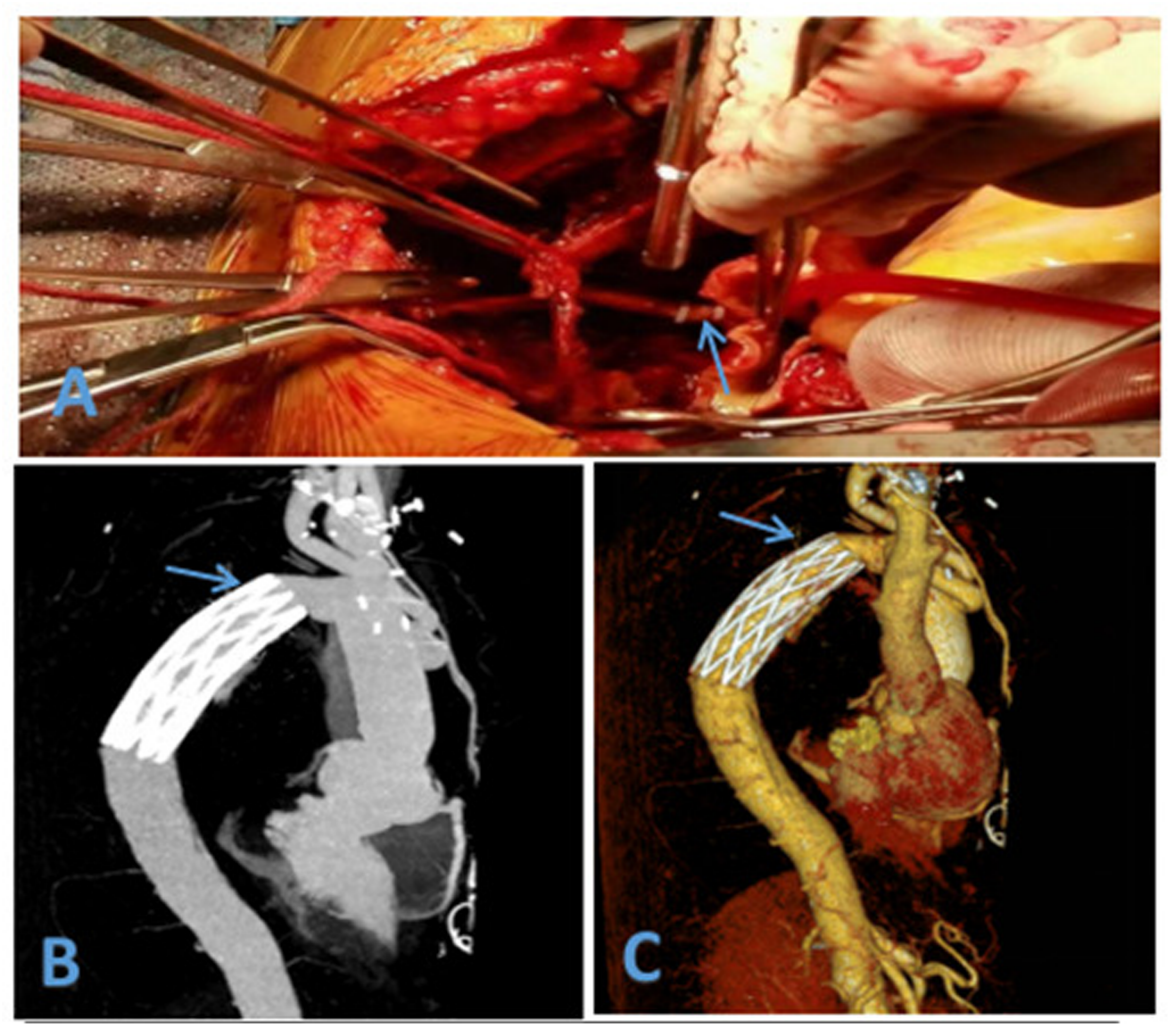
(A) Hem-o-lok vascular clip used to close the LSA (B) postoperative CTA (C) postoperative CTA (3D).
